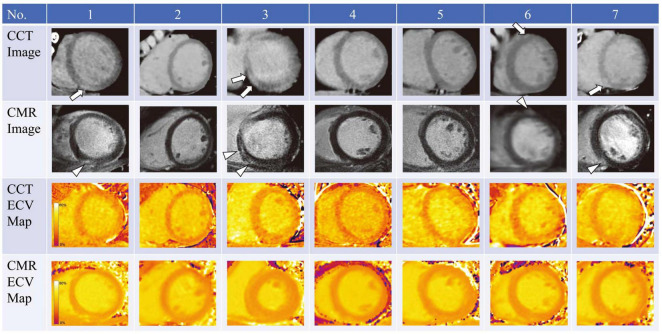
Cardiac computed tomography (CCT) and cardiac magnetic resonance (CMR) imaging in 7 patients who underwent anthracycline treatment. Arrows and arrowheads indicate late iodine and gadolinium enhancements, respectively. ECV, extracellular volume. Note: this image is reproduced with permission from Sueta et al. (74).
